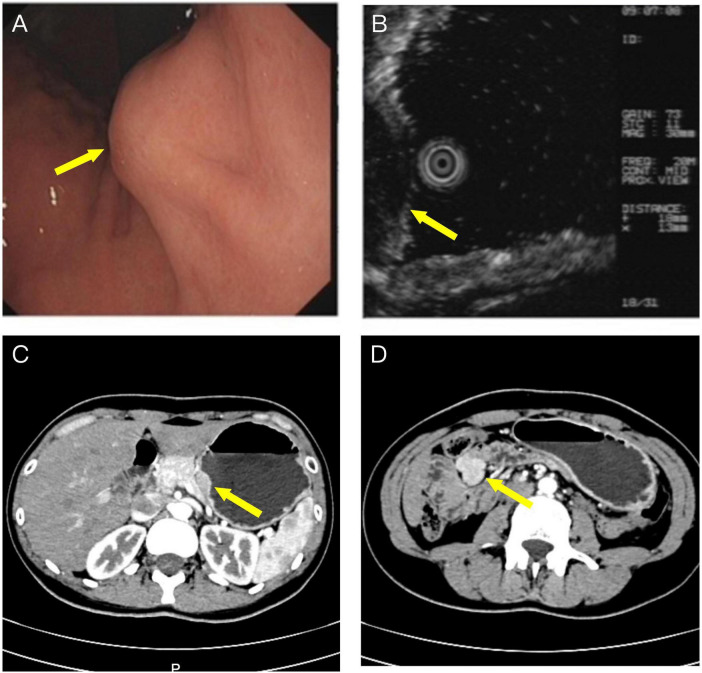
Preoperative endoscopic and imaging findings in Case 1. (A) Gastroscopy revealed a submucosal protruding lesion in the gastric body with a smooth surface. (B) Endoscopic ultrasonography demonstrated a hypoechoic lesion measuring approximately 1.8 × 1.3 cm arising from the muscularis propria. (C) Contrast-enhanced computed tomography (CT) showed a markedly enhancing nodule measuring approximately 16–17 mm along the lesser curvature of the stomach. (D) Contrast-enhanced CT revealed an approximately 10-mm enhancing nodule in the small intestine. Arrows indicate the lesions.
